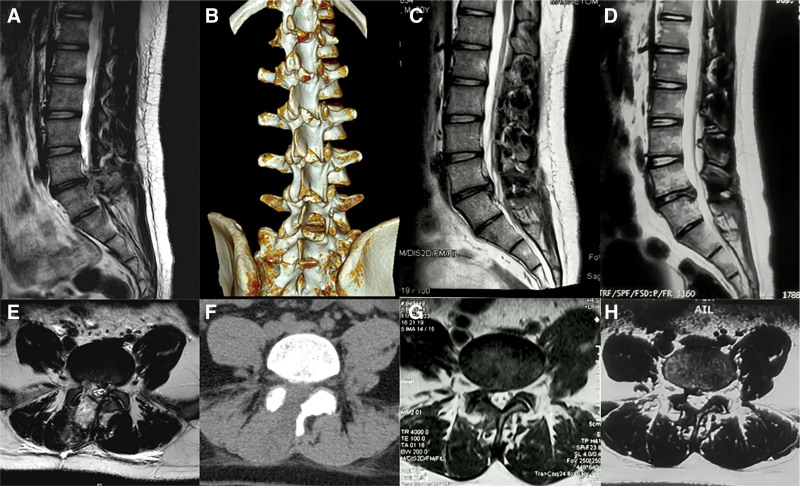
Postoperative imaging. Postoperative sagittal (A) and axial (L) MRI T2-weighted images obtained 1 week after surgery, demonstrating successful decompression at L5/S1 with preserved ligamentum flavum and proper spinal alignment. Postoperative sagittal (B) and axial (F) CT images obtained 1 week after surgery, confirming proper decompression and structural integrity at L5/S1 without any residual bone fragments or compression. Postoperative sagittal (C) and axial (G) MRI T2-weighted images obtained 1 month after surgery, showing continued maintenance of decompression at L5/S1 with no recurrence of disc herniation and clear nerve root pathways. Postoperative sagittal (D) and axial (H) MRI T2-weighted images obtained 6 months after surgery, indicating stable decompression at L5/S1, with no recurrence of herniation and clear neural structures. CT = computed tomography, MRI = magnetic resonance imaging.
